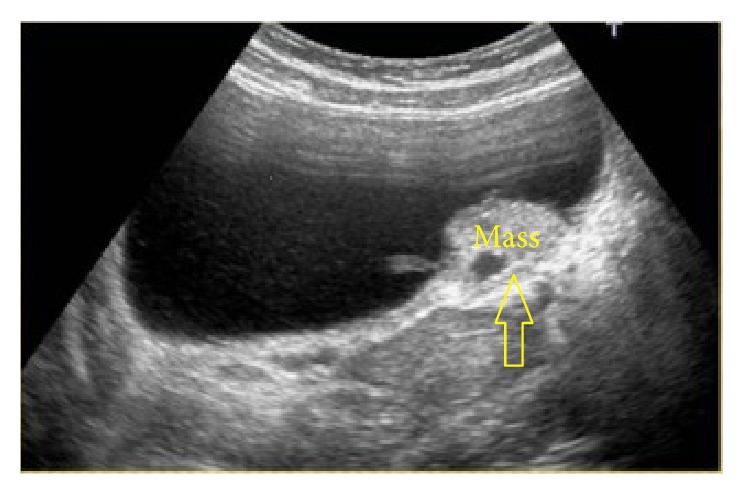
Urinary system ultrasonography showing a massive lesion of 3 × 4 cm extending into the bladder on the left side wall of the bladder.
